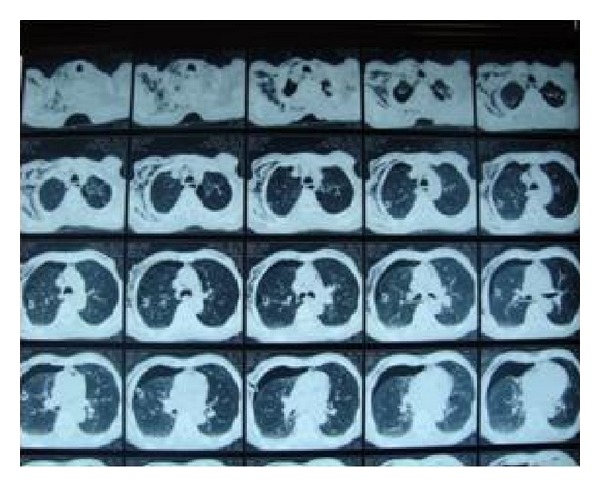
Thorax CT: bullous areas at the apexes of both lungs and a right pneumothorax.
